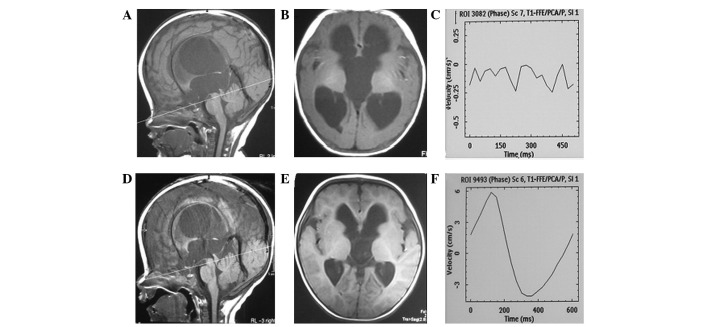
(A) Sagittal and (B) coronal magnetic resonance imaging (MRI) prior to neuroendoscopic aqueductoplasty (EAP); (C) Cerebrospinal fluid (CSF) flow velocity at the aqueduct fistula level measured by phase-contrast MRI prior to EAP. (D) The postoperative image demonstrates a patent aqueduct with a flow void. (E) Scan following EAP revealed reduced ventricular size, cephalus and membranous obstruction with prestenotic dilatation. (F) CSF flow velocity at the aqueduct fistula level measured by phase-contrast MRI following aqueductoplasty. Quantification of CSF flow was performed in the transverse plane perpendicular to the midcollicular level.
